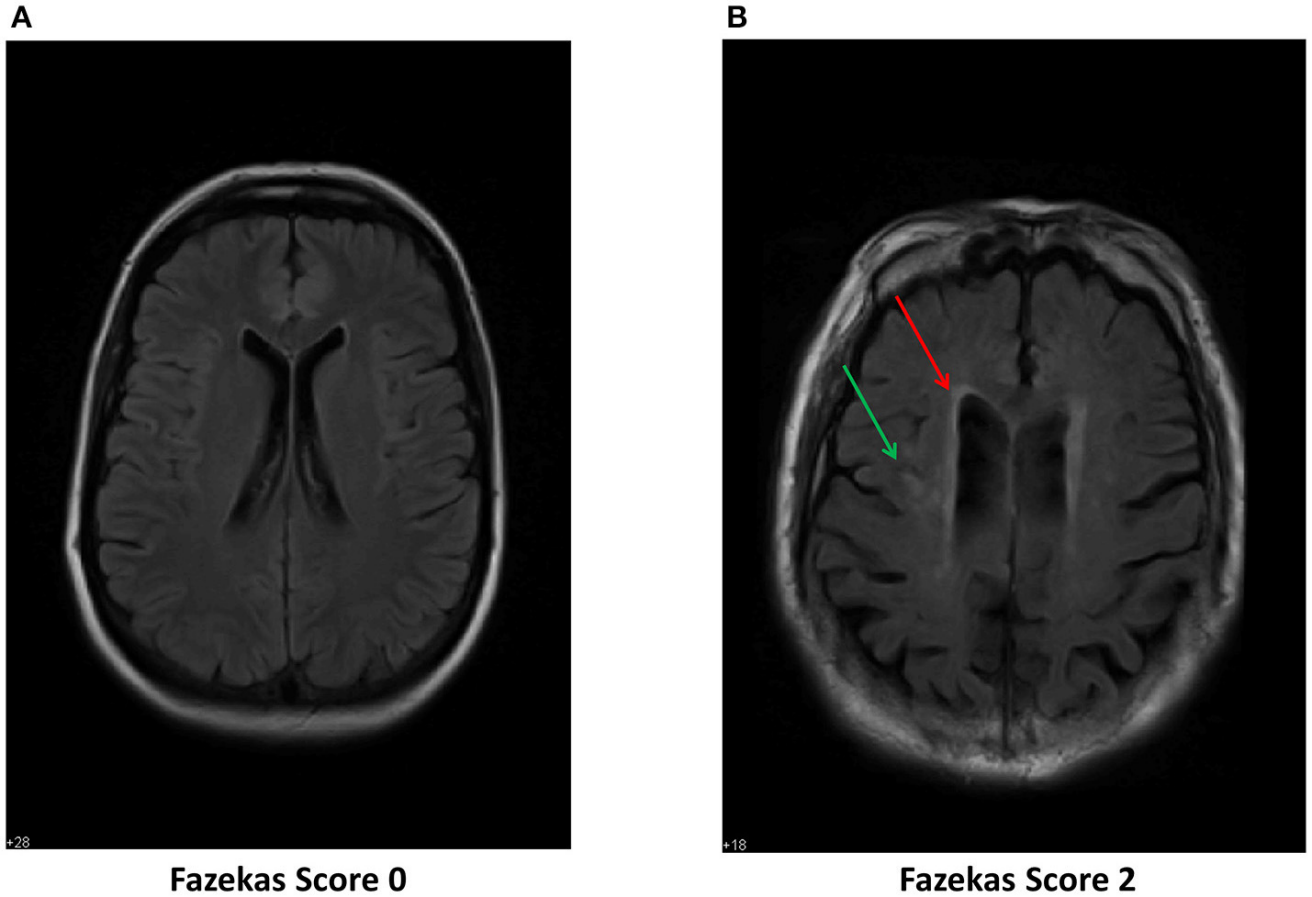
Axial FLAIR images of two of the study patients. (A) Shows normal white matter imaging. This patient was scored Fazekas Grade 0. (B) Shows areas of hyperintensity in the periventricular regions (red arrow) and in the deep white matter (green arrow). This signature has been identified as a marker for leucoaraiosis. This image was blindly scored and on neuro-radiology report identified as Fazekas Grade 2.
